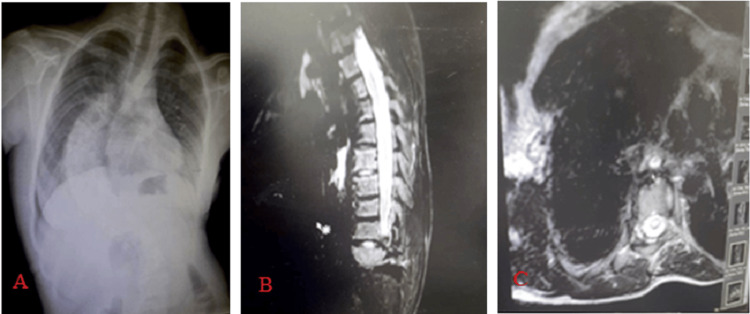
A fifteen-year-old female AIS patient with syrinx seen at C6–C7 on preparative MRI. A: Chest X-ray showing scoliosis, B: T2 sagittal (cervical and thoracic) showing C6-C7 syrinx, C: axial T2 C6-C7 syrinx AIS: Adolescent idiopathic scoliosis
